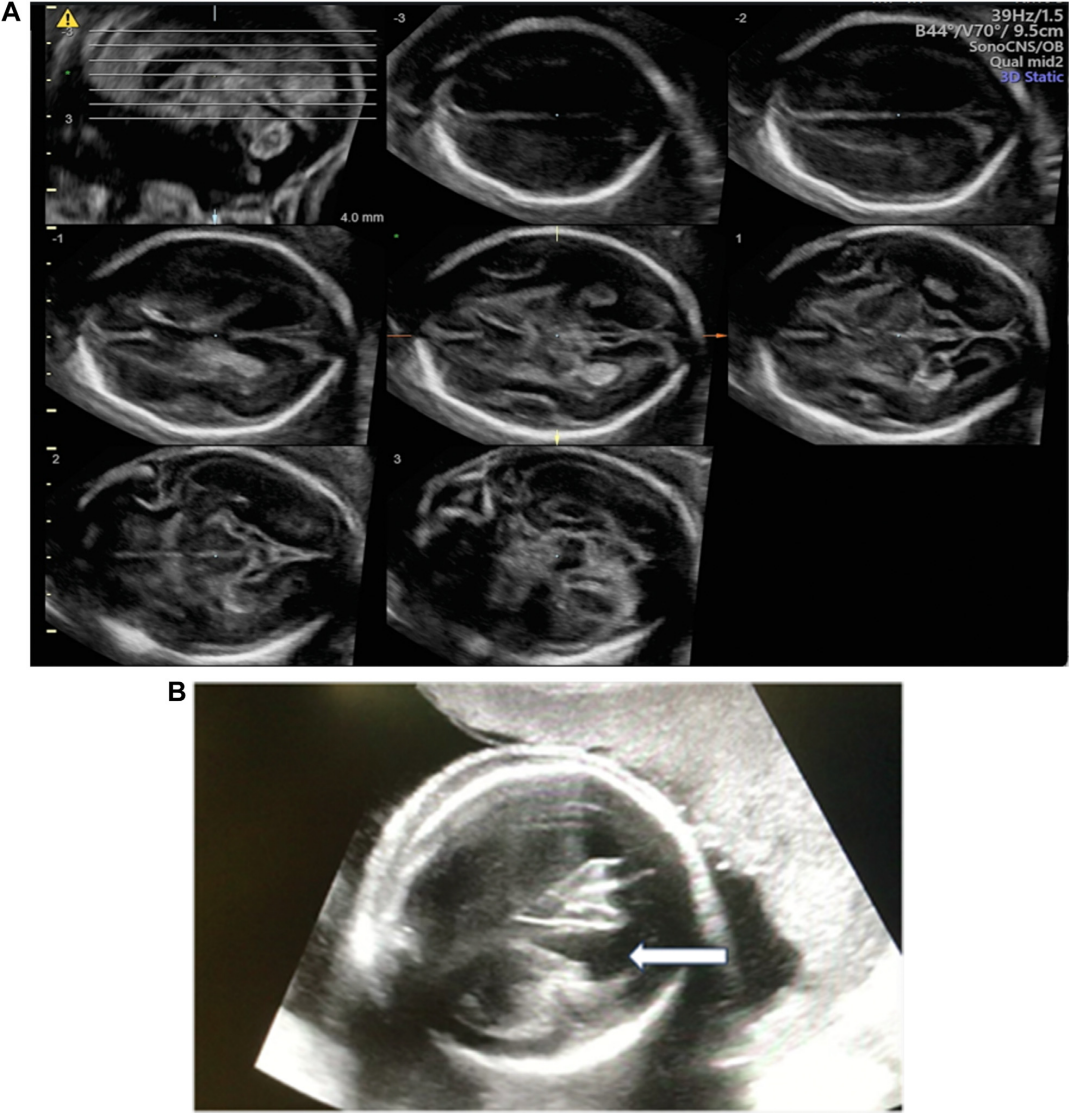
Fetal Neurosonography (A) Fetal neurosonography in transverse planes showing normal findings in our case at 23+0 weeks of gestation. (B) For comparison, an exemplary case of fetal ventriculomegaly (white arrow) due to hypotension and ischemia in a patient on extracorporeal membrane oxygenation during severe acute respiratory syndrome coronavirus 2 infection; transverse plane, 23+5 weeks of gestation.
